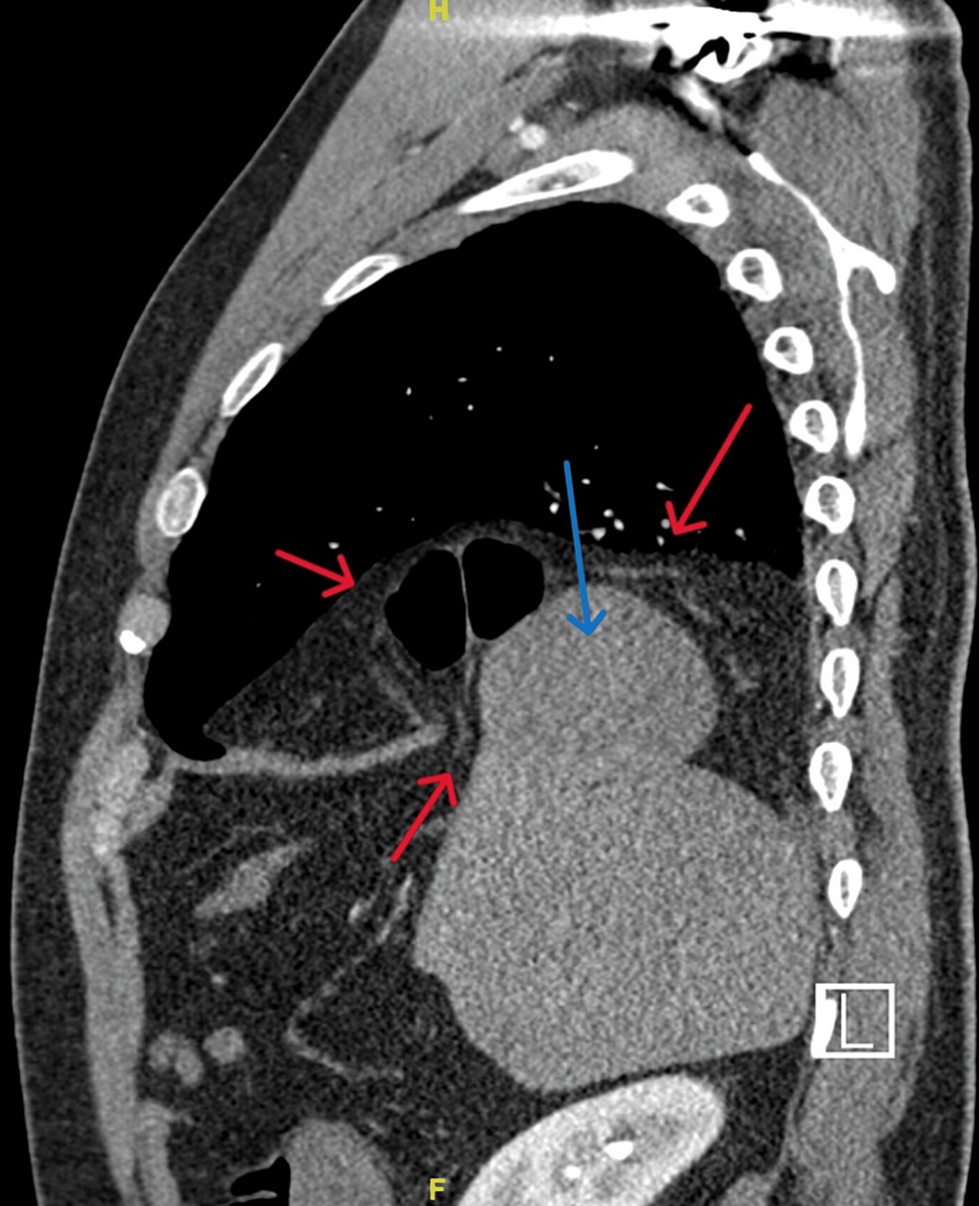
Sagittal chest/abdomen CT showing right diaphragmatic hernia with Chilaiditi sign. Red Arrows: Large Bowel, Blue Arrows: Liver
